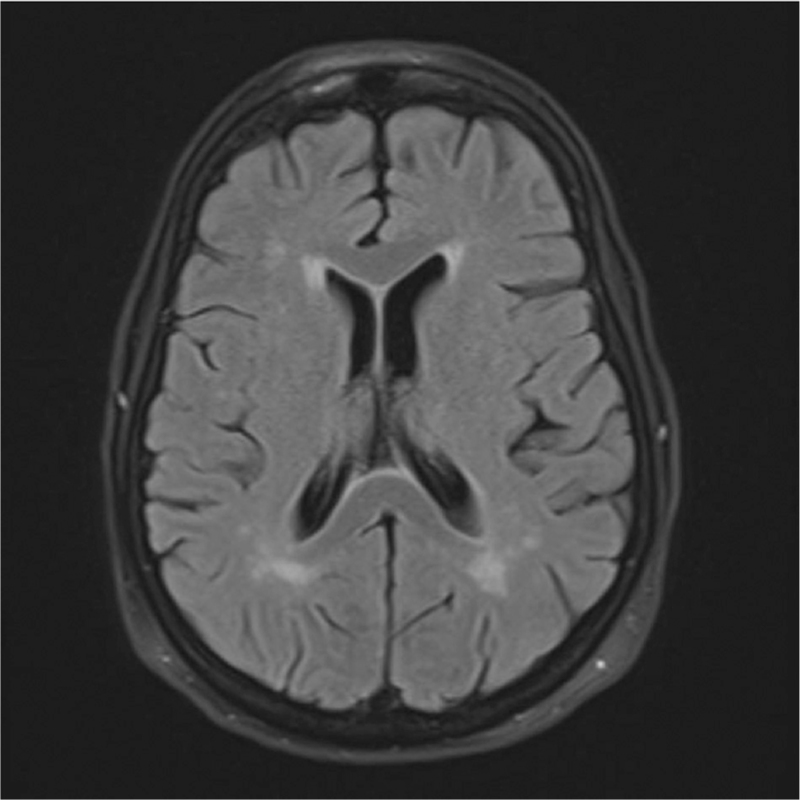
Axial FLAIR MRI acute infarcts bilaterally across corona radiata.
